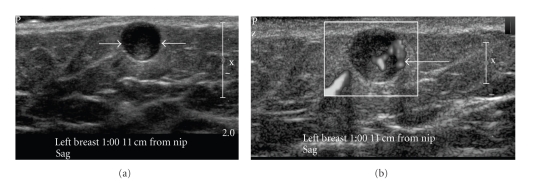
Ultrasound with a 17 MHZ linear probe. (a) demonstrates a 7 mm well-defined, complex hypoechoic mass within the breast superficially, abutting the skin (white arrows). (b) The mass shows significant central vascularity.
